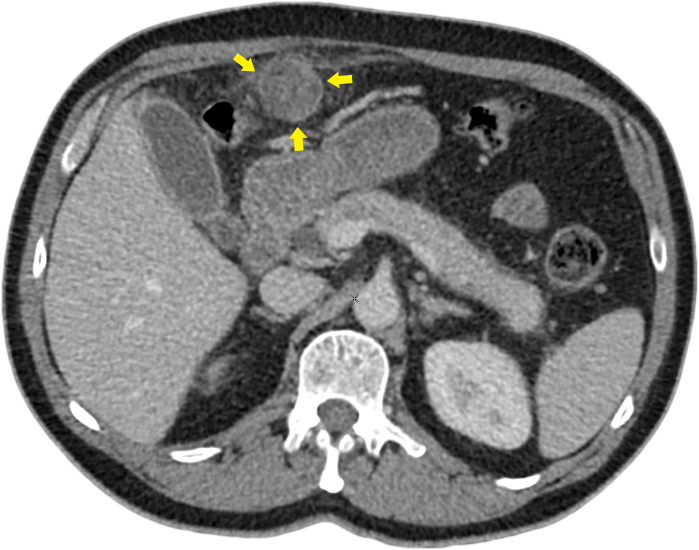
Intravenous contrast-enhanced (portal venous phase) multidetector CT axial image showing the thrombosed paraumbilical vein (yellow arrows), which measures 3.8 cm in maximal dimensions. No identifiable internal flow was evident either on the arterial phase or the portal venous phase.
